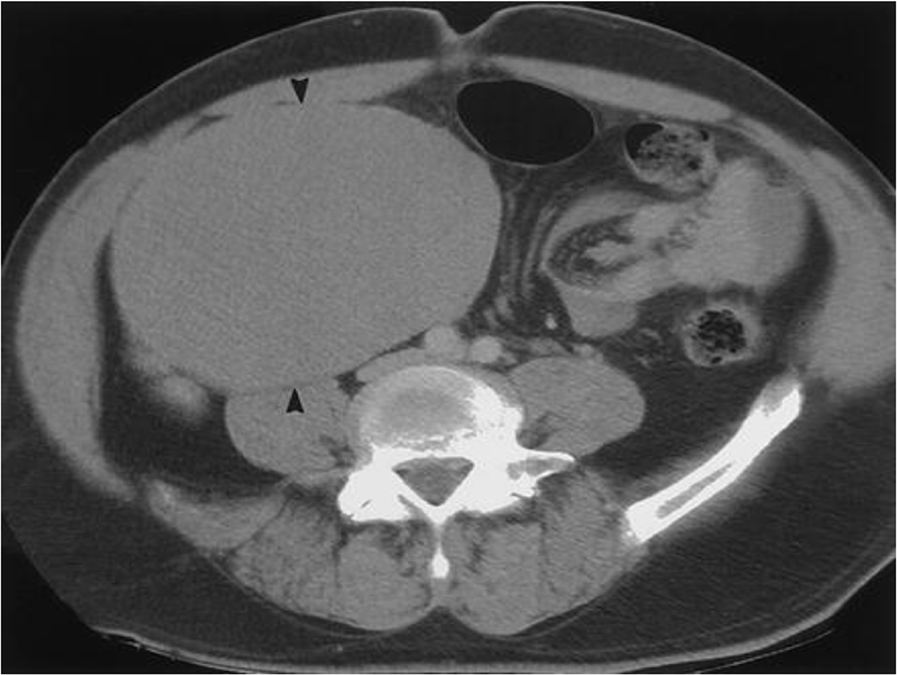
CT image of the abdomen shows a large retroperitoneal pelvic mass.
